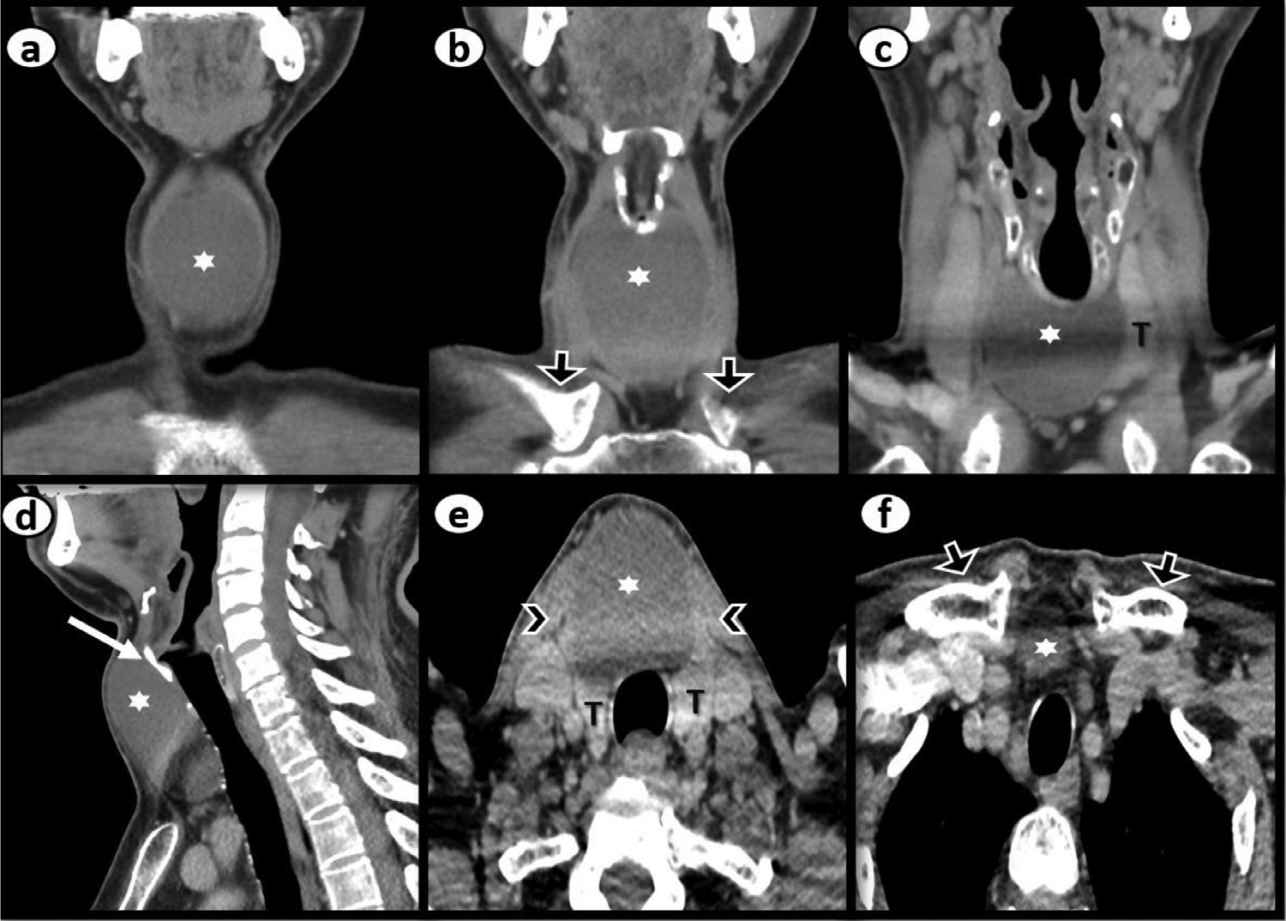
(a-f): Contrast enhanced computed tomography (CECT) neck: Coronal images showing colloid cyst (asterisks) from anterior to posterior (a-c). Sagittal image (d) showing extent of the cyst from thyroid cartilage (arrow) to just above the clavicles (open arrows b,f). Axial image (e) showing relationship between cyst (asterisk) and thyroid gland (T), note the anterolateral displacement of strap muscles (arrowheads).
